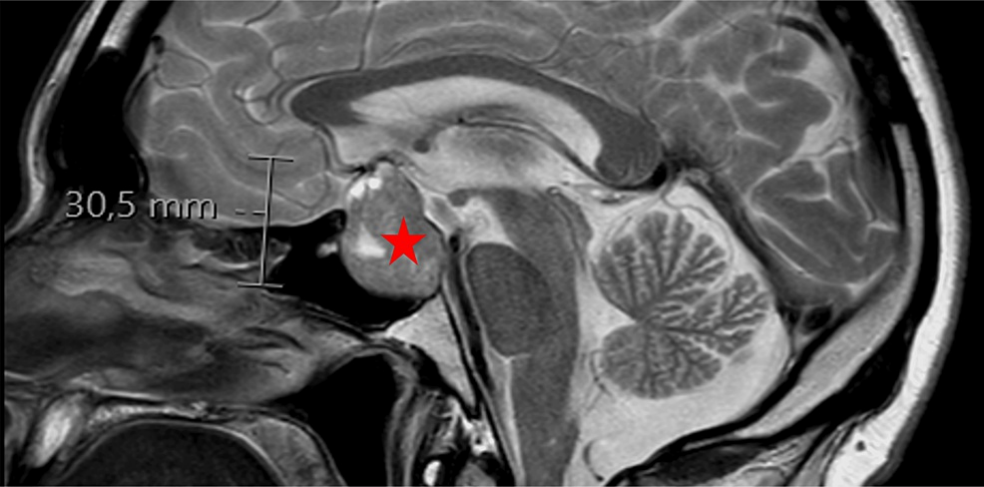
MRI showing a hypophyseal macroadenoma T2W MRI sagittal section displaying a 30.5 mm hypophyseal macroadenoma (red star) with suprasellar extension grade 3 according to SIPAP grading. Signs of erosion are evident in the posterior wall of the sella, and the chiasma is elevated by the adenoma MRI: magnetic resonance imaging
